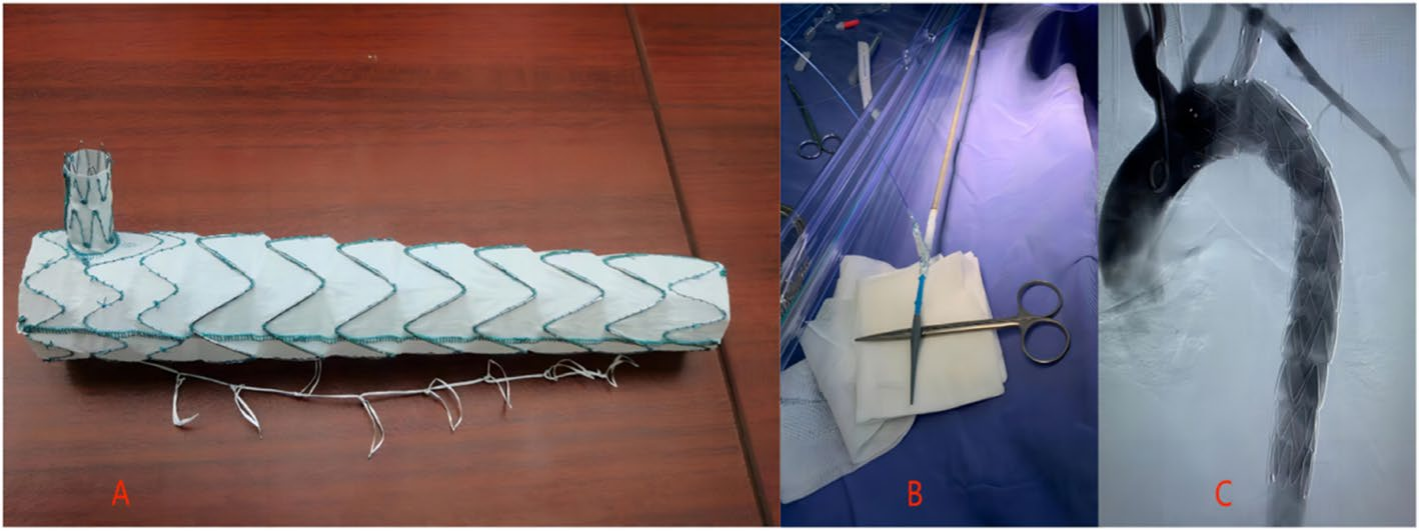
Picture A: Castor integrated stent physical image. Picture B: Pre-release image of the castor integrated stent. Picture C: Post-release radiographic image of the castor integrated stent
